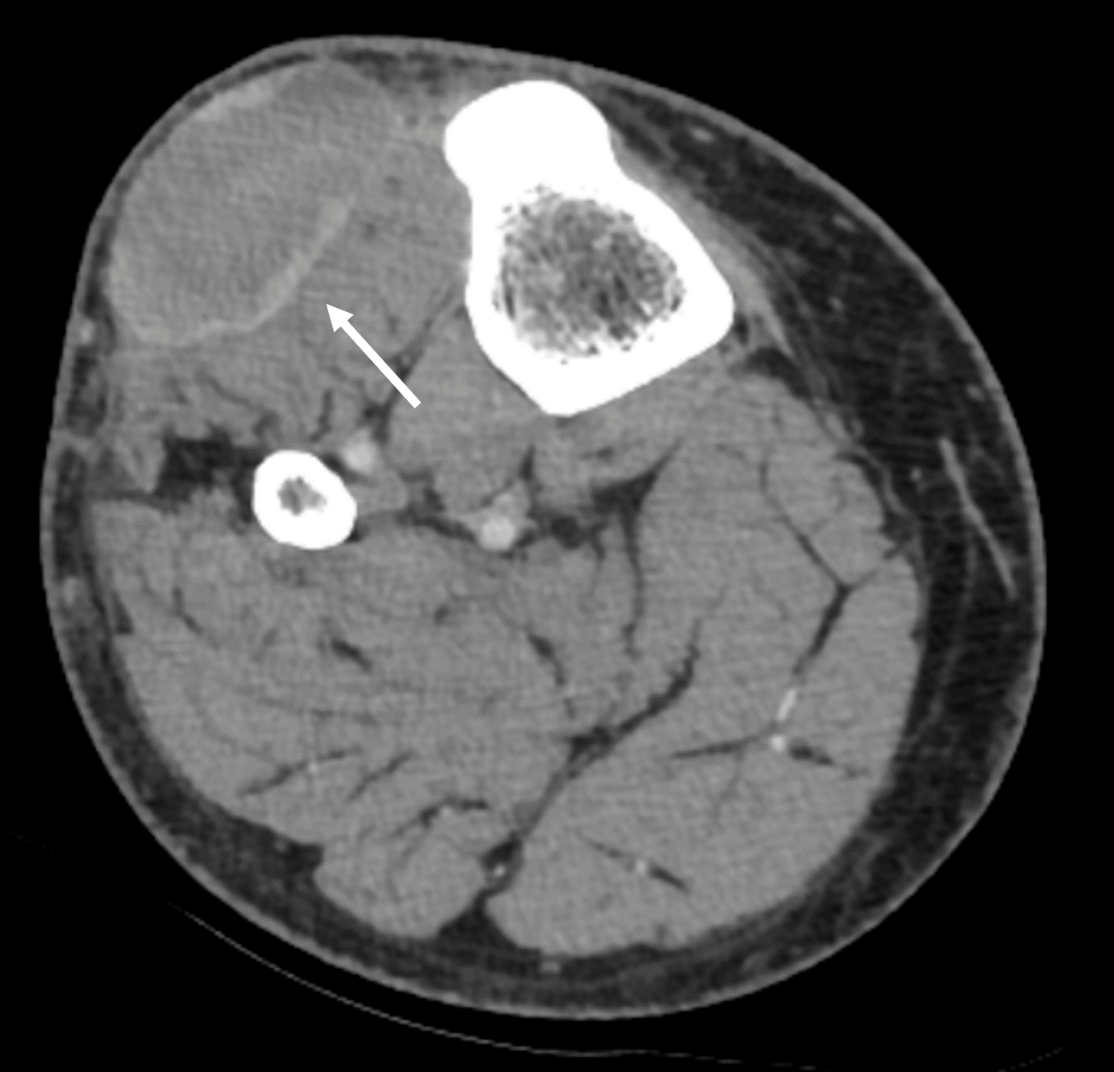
Contrast-enhanced CT of the right lower extremity 8.2 × 1.6 × 5.7 cm mass-like area (white arrow) with central fluid attenuation, septations, and peripheral enhancement located in the soft tissues lateral to the proximal tibia within the subcutaneous tissues overlying the musculature. There is sparing of the adjacent tibial cortex.
